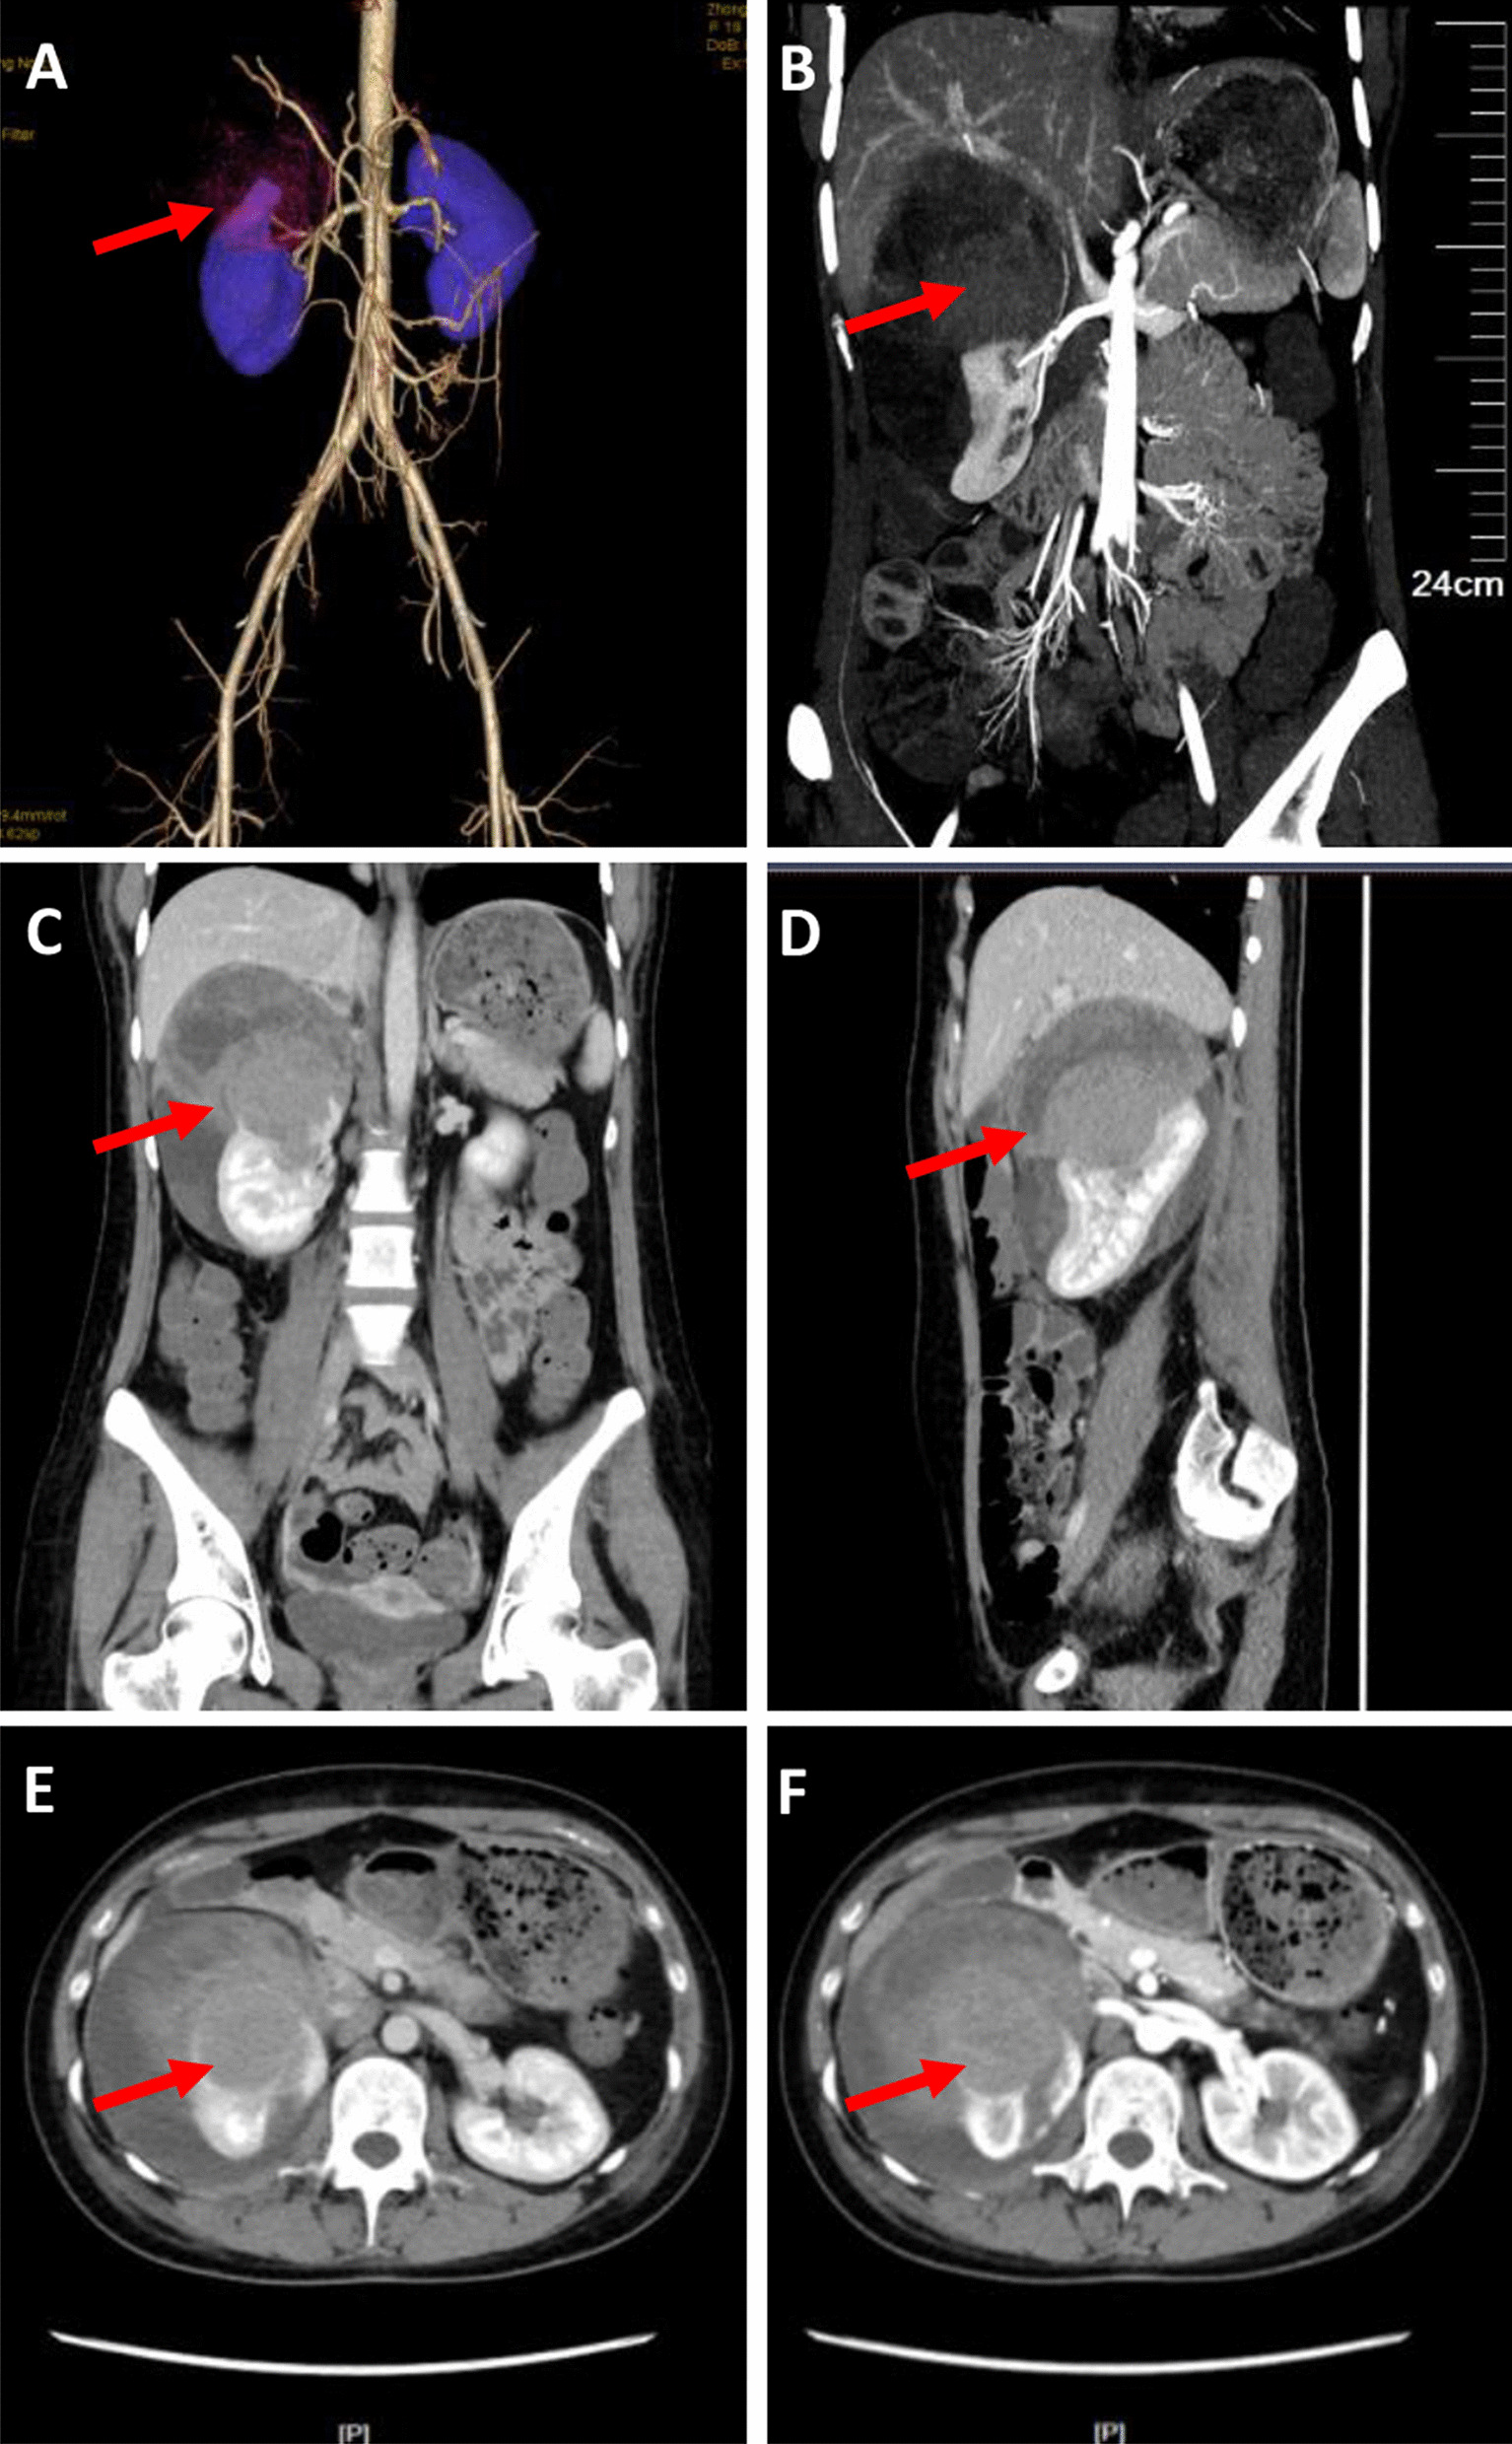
Abdominal imaging with contrast-enhanced computed tomography and computed tomography angiography images. A, B Computed tomography angiography images; C–F Abdominal imaging with contrast-enhanced computed tomography images
